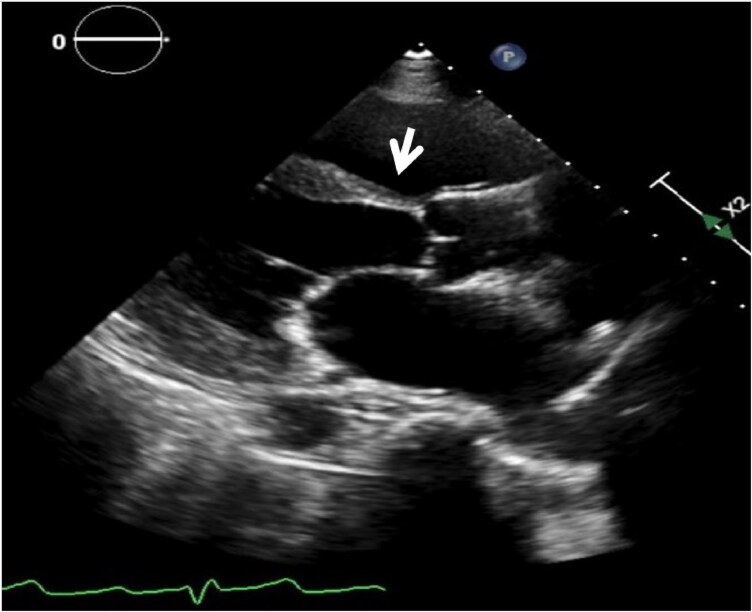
Transthoracic echocardiogram shows thinning of the basal segment of the interventricular septum.
